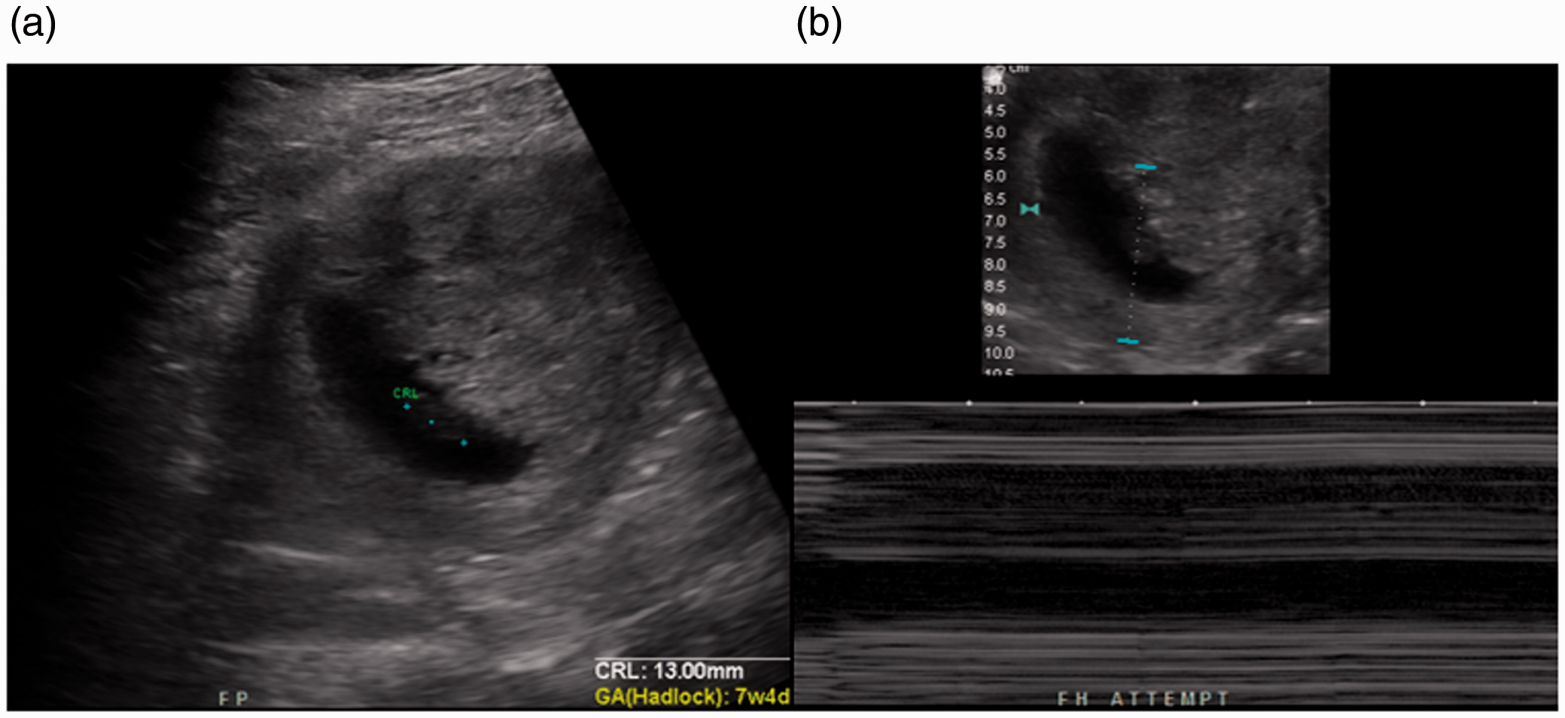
(a) Ultrasound image generated using the telerobotic ultrasound system
